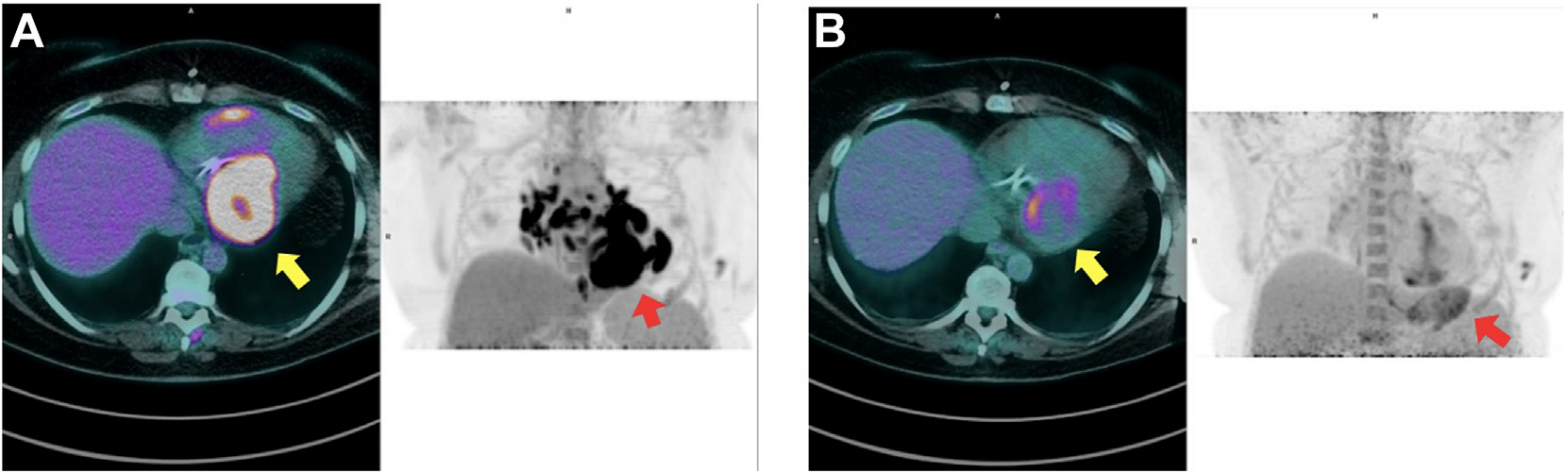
PET/CT at baseline (A) and at 4-month follow-up after immunosuppressive treatment (B) using 2-[^18^F]-fluorodeoxyglucose, axial displays at the level of the heart *(left, color figures)* and coronal whole-body displays *(right, black and white figures)*. Panel A demonstrates diffuse and patchy metabolic activity particularly involving the IVS, inferior LV wall, and right ventricular free wall, as well as multiple foci of intense metabolic activity involving mediastinal lymph nodes and less intense activity in pulmonary nodules and the hilum; panel B shows partial metabolic response in the heart with minimal residual metabolic activity in the inferior basal LV wall (maximum standardized uptake value 7 vs 18) and complete metabolic response of the mediastinal lymph nodes and lung nodules.
